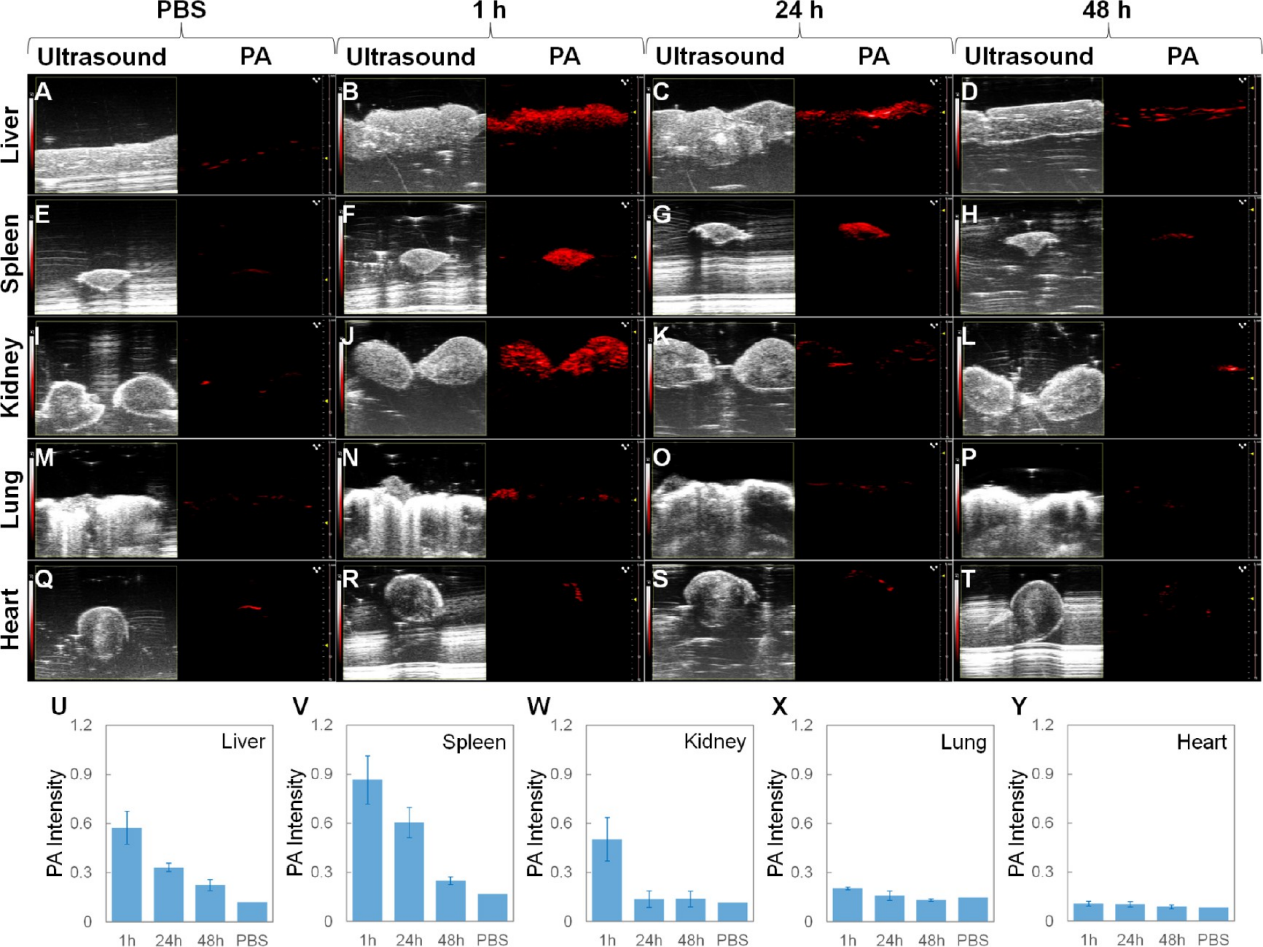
(A–T) Time-dependent ultrasound/PA images and (U–Y)
